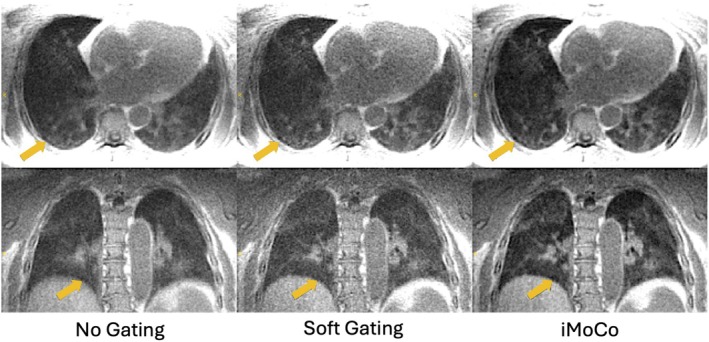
Sample datasets with differing clinical interpretation between readers. Images in each column show representative axial (top) and coronal (bottom) slices from three UTE motion mitigation schemes, acquired in a patient classified as possible UIP pattern from CT imaging. Two readers scored the “no gating” dataset as “unknown,” while the third reader scored it as “possible IPF.” With the soft‐gating reconstruction, the scores were reversed, with the first two readers scoring it as “possible IPF,” while the third reader scored it as “unknown.” With the improved contrast and visualization of regional patterns (examples shown in yellow arrows) from iMoCo, all readers agreed on a clinical classification of “possible IPF,” matching the CT designation.
